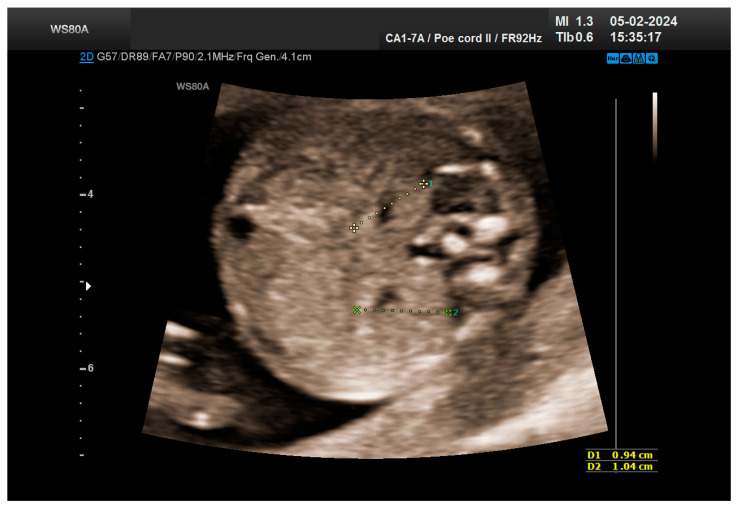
The two kidneys are clearly visualized: the right one, ectopic, fused with the left kidney, normally positioned—ultrasonography revaluation at 17 weeks.
